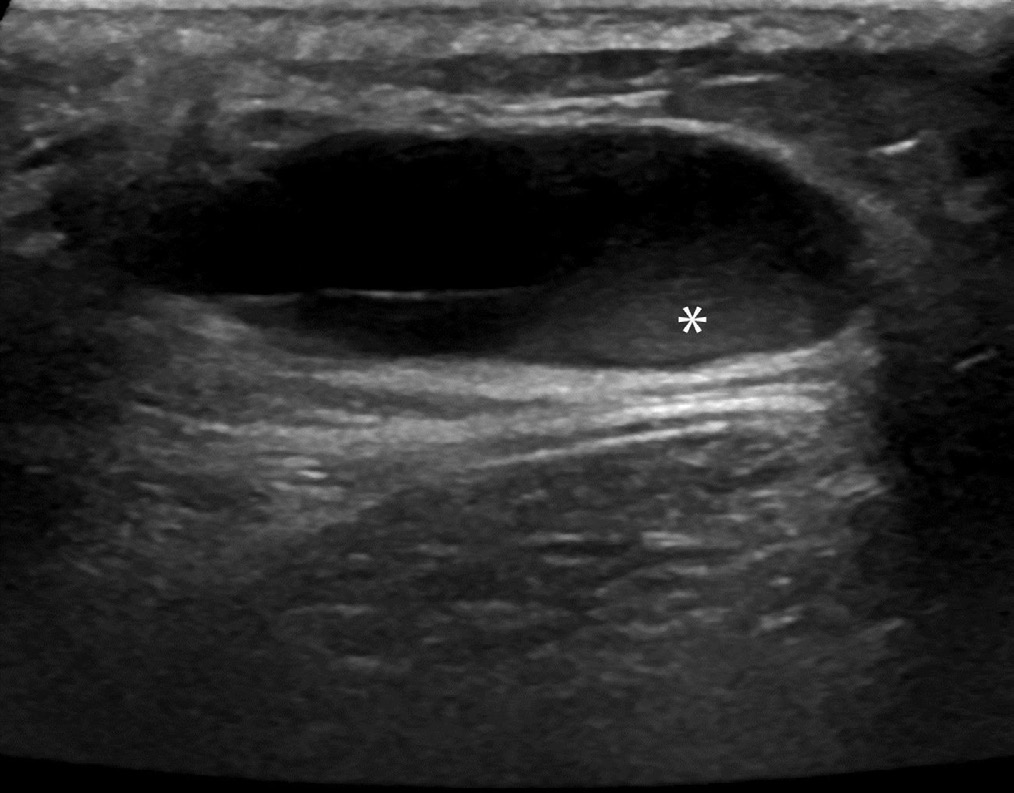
Transverse sonographic image of the upper back shows a predominantly anechoic subcutaneous lesion with posterior acoustic enhancement containing a small soft tissue nodular component (*).
